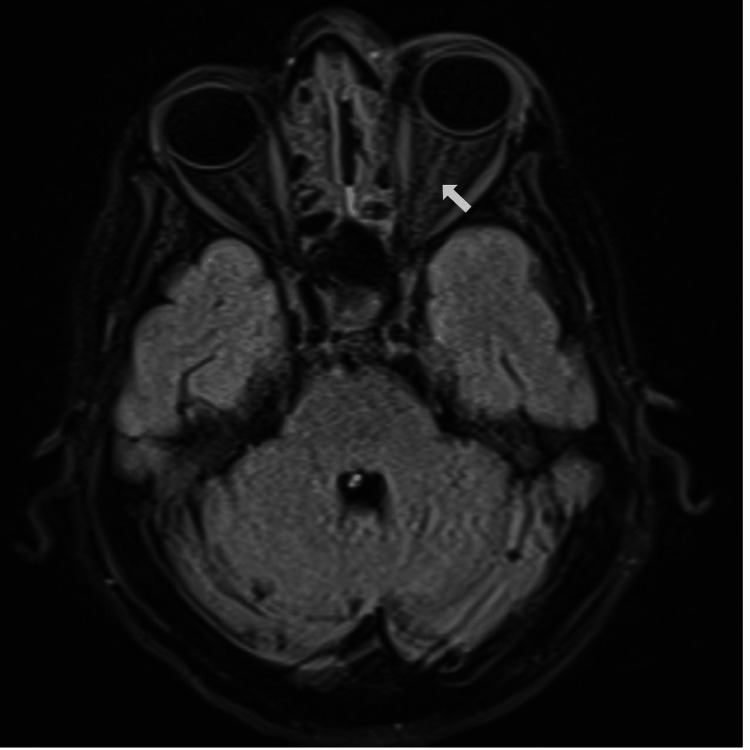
Axial section of a bilateral non-contrast orbital MRI at the patient's initial hospital admission showing increased inflammatory T2 signal around the left optic nerve (white arrow).
